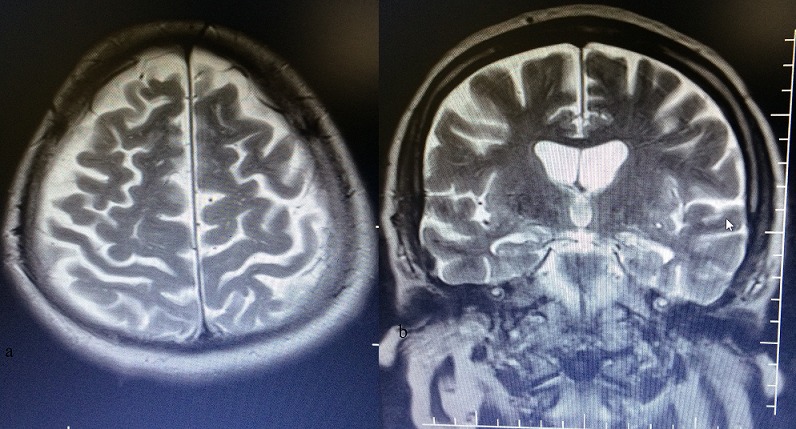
Follow-up MRI scans 1 month later showed spontaneous resolution of bilateral subdural hematoma
